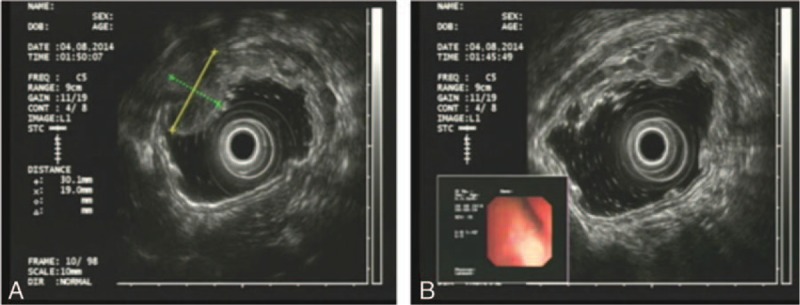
EUS revealed obvious thickening of the submucosa and muscularis propria with hypoechoic lesion (A), lesions with multiple hypoechoic and luminal structure (B) of various sizes in 1 patient of gastric vascular malformations. EUS = endoscopic ultrasonography.
